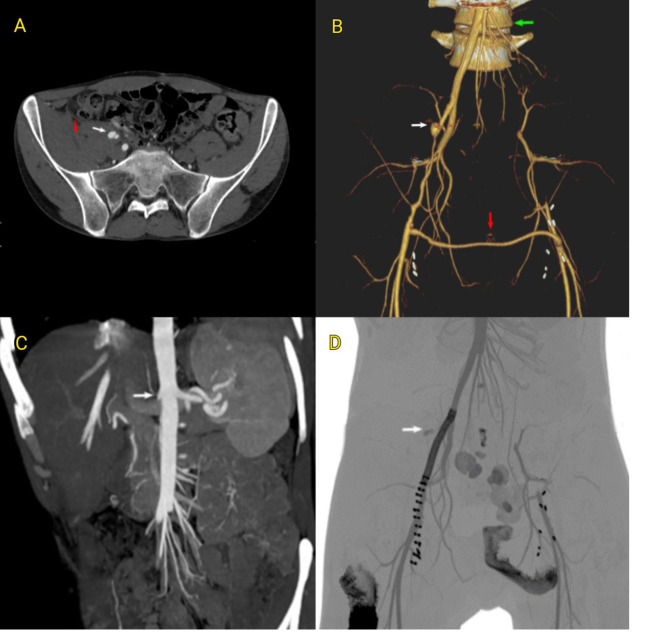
(A) Computed tomography (CT) angiography, axial plane, revealed a dissected right external iliac artery (EIA) with a pseudoaneurysm (white arrow) and an enlarged, hemoraginous right iliopsoas muscle (red arrow). (B) CT angiography, 3D Volume Render (VR) image shows a narrowed true lumen of the right EIA with a pseudoaneurysm and occluded false lumen (white arrow). Patent femorofemoral bypass (red arrow). Pelvic collaterals due to a previously ligated, ruptured left common iliac artery (green arrow). (C) CT angiography (maximum intensity projection) shows a stump of the right renal artery after nephrectomy due to a rupture of renal artery aneurysm. (D) CT angiography, VR image shows an implanted stent graft in the right EIA (white arrow). Postinterventional occlusion of the femorofemoral bypass is revealed.
